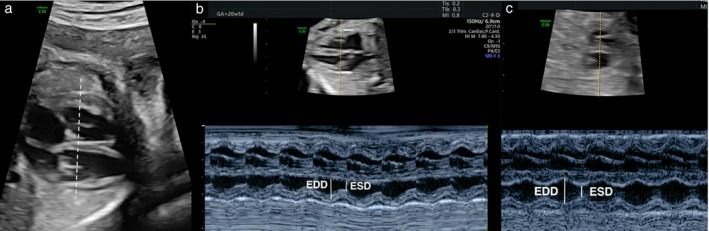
Assessment of ventricular shortening fraction (SF). (a) Optimized four‐chamber view with adjustment of two‐dimensional image depth and magnification so that the fetal heart occupies more than two‐thirds of the image sector. Plane of the M‐mode cursor passes through the right ventricular free wall, ventricular septum and left ventricular free wall perpendicular to their plane and just below the mitral valve annulus. (b) The four‐chamber M‐mode tracing acquired demonstrates multiple cardiac cycles. The end‐diastolic diameter (EDD) is measured as the largest diameter of the ventricle, shown here in the left ventricle, and the end‐systolic diameter (ESD) as the smallest diameter. SF (%) is calculated as ((EDD − ESD)/EDD) × 100. (c) From a four‐chamber view with the ventricular septum and free walls perpendicular to the ultrasound beam, the transducer is rotated 90°, and an M‐mode tracing through the ventricular short axis at the level of the ventricular papillary muscles can also be used to assess SF. Dashed line indicates M‐mode cursor.
